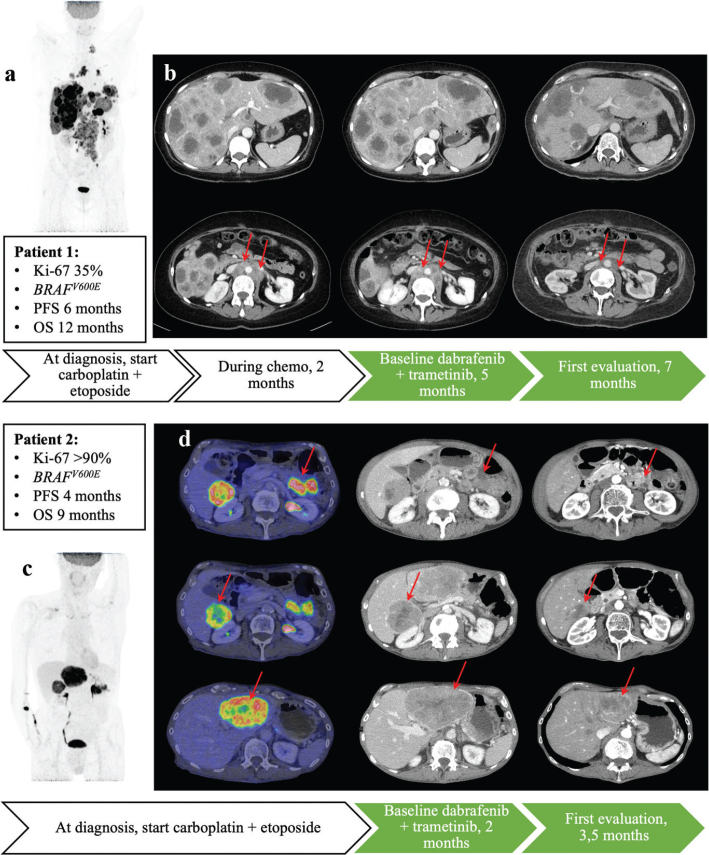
Patients having received genetics-guided therapy 2018–2023. (a) Fludeoxyglucose positron emission tomography (FDG-PET) image (Maximum Intensity Projection, MIP) of patient 1 before starting chemotherapy following surgery. (b) Patient 1’s CT images before and during treatment with dabrafenib and trametinib. First row: liver metastases. Second row: nodal metastases. (c) Fludeoxyglucose positron emission tomography (FDG-PET) image (MIP) of patient 2 before starting chemotherapy. (d) Patient 2’s FDG-PET/CT and CT images (PET/CT left, CT middle and right) before and during treatment with dabrafenib and trametinib. First row: primary tumor. Second row: right liver metastasis. Third row: left liver metastasis. PFS = progress-free survival. OS = overall survival.
